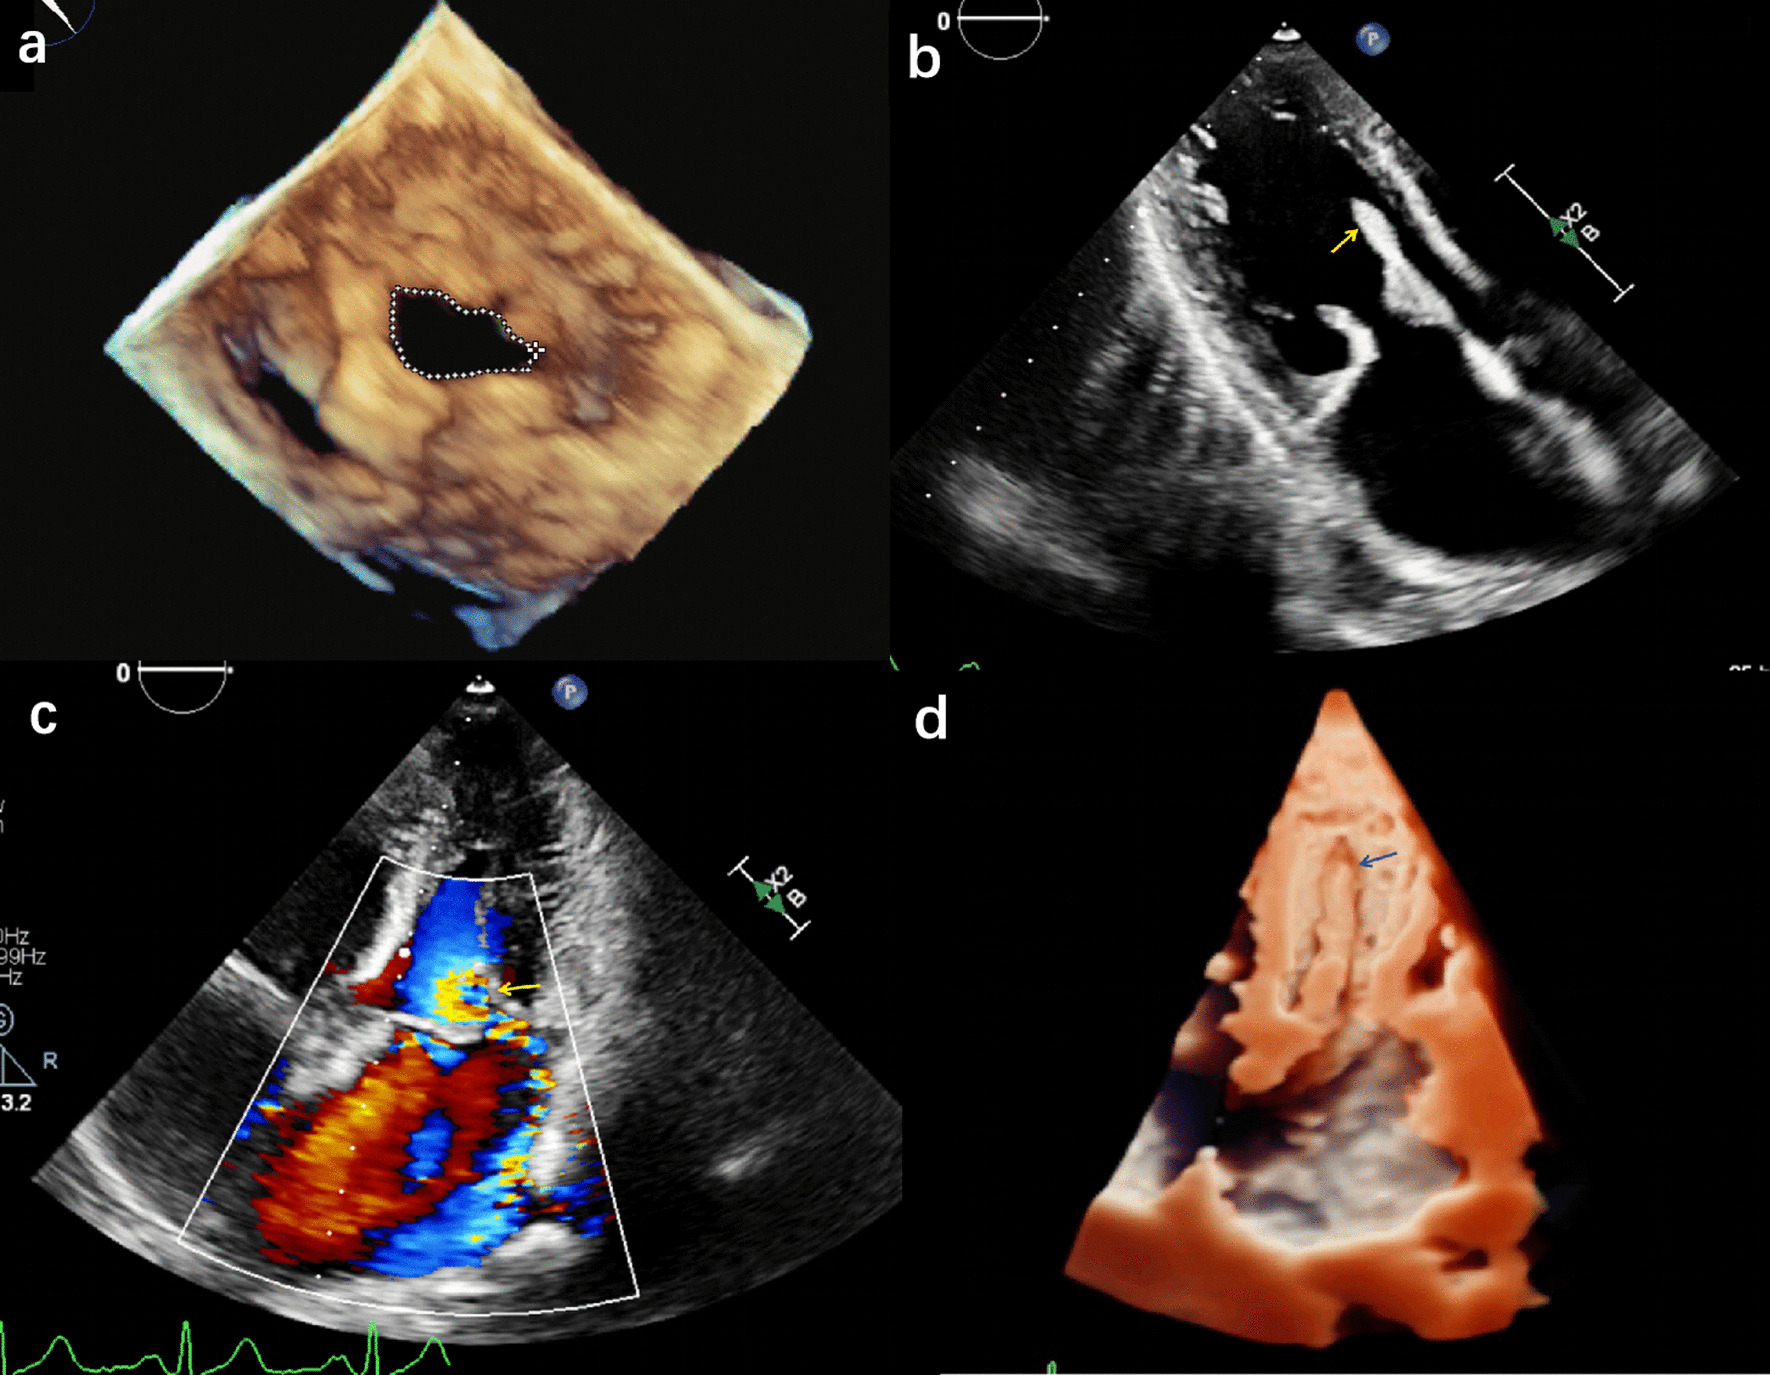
a Three-dimensional transesophageal echocardiography revealing mitral stenosis. b Transthoracic echocardiography revealing avulsion of the papillary muscle from the left ventricular wall. c Color Doppler Flow Imaging revealing severe mitral regurgitation (yellow arrow). d Three-dimensional echocardiogram revealing papillary muscle avulsion (blue arrows)
